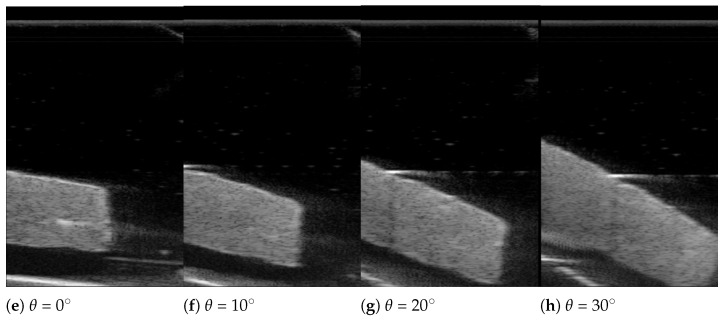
Images during ultrasound scanning.
